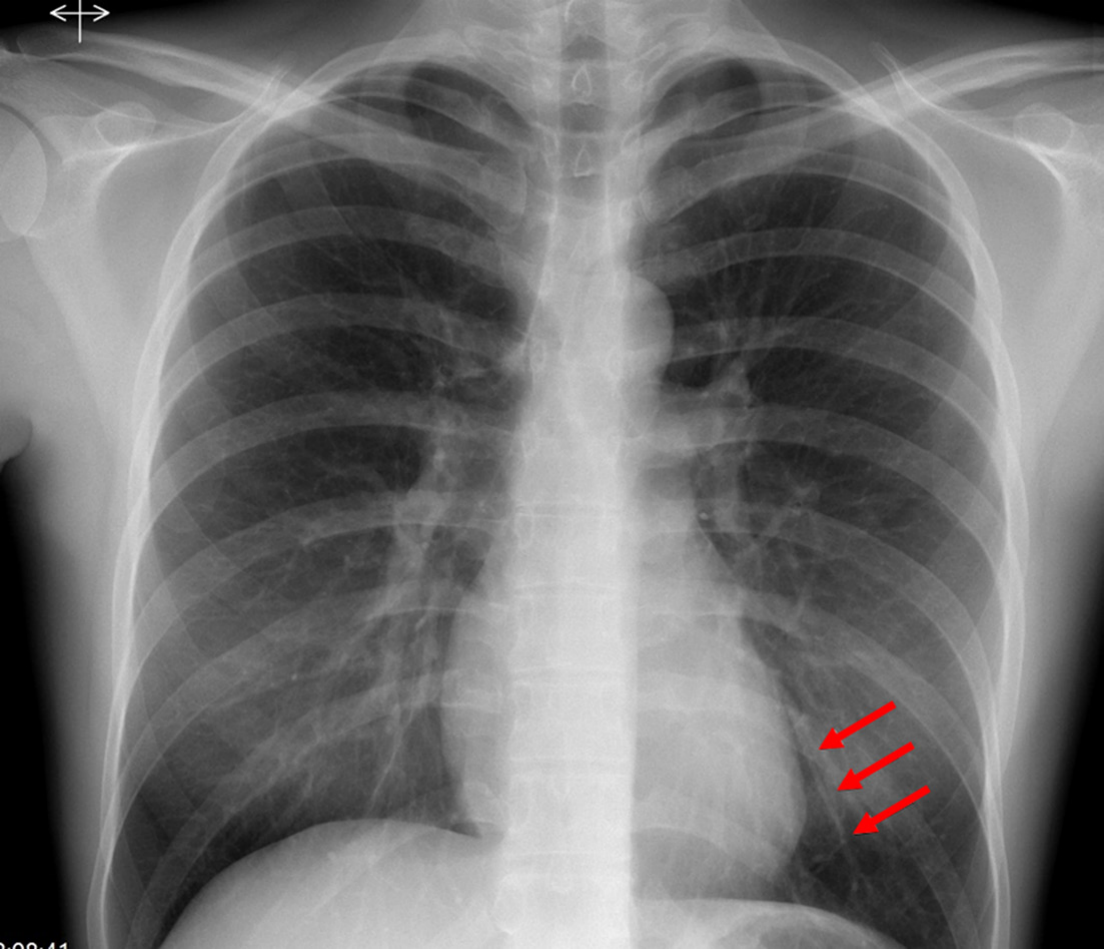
Chest X-ray with arrows demonstrating 4 cm linear opacity in the left lower zone.
